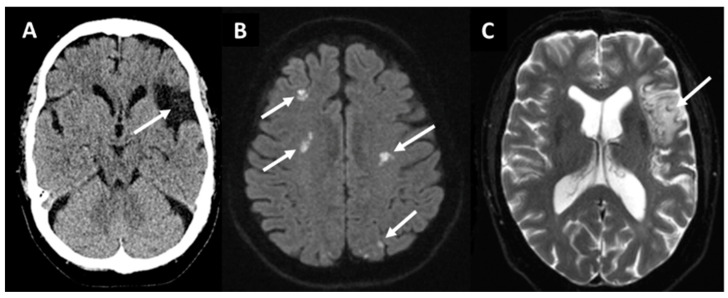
Non-contrast computed tomography (A) showing a chronic embolic stroke of undetermined source (arrow) in a 66-year-old woman in the left middle cerebral artery territory. Magnetic resonance diffusion-weighted imaging (B) revealing ischemic lesions (bright spots indicated by arrows) in multiple territories in a 78-year-old man with chronic atrial fibrillation. T2*-weighted imaging (C) showing a subacute lesion (arrow) in the left middle cerebral artery territory in a patient with paroxysmal atrial fibrillation.
